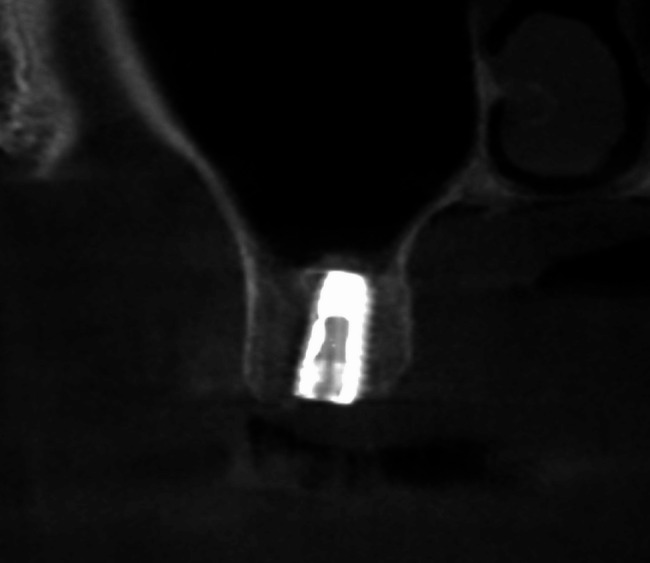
CBCT after 6 months of endoscopic assisted crestal sinus lifting
